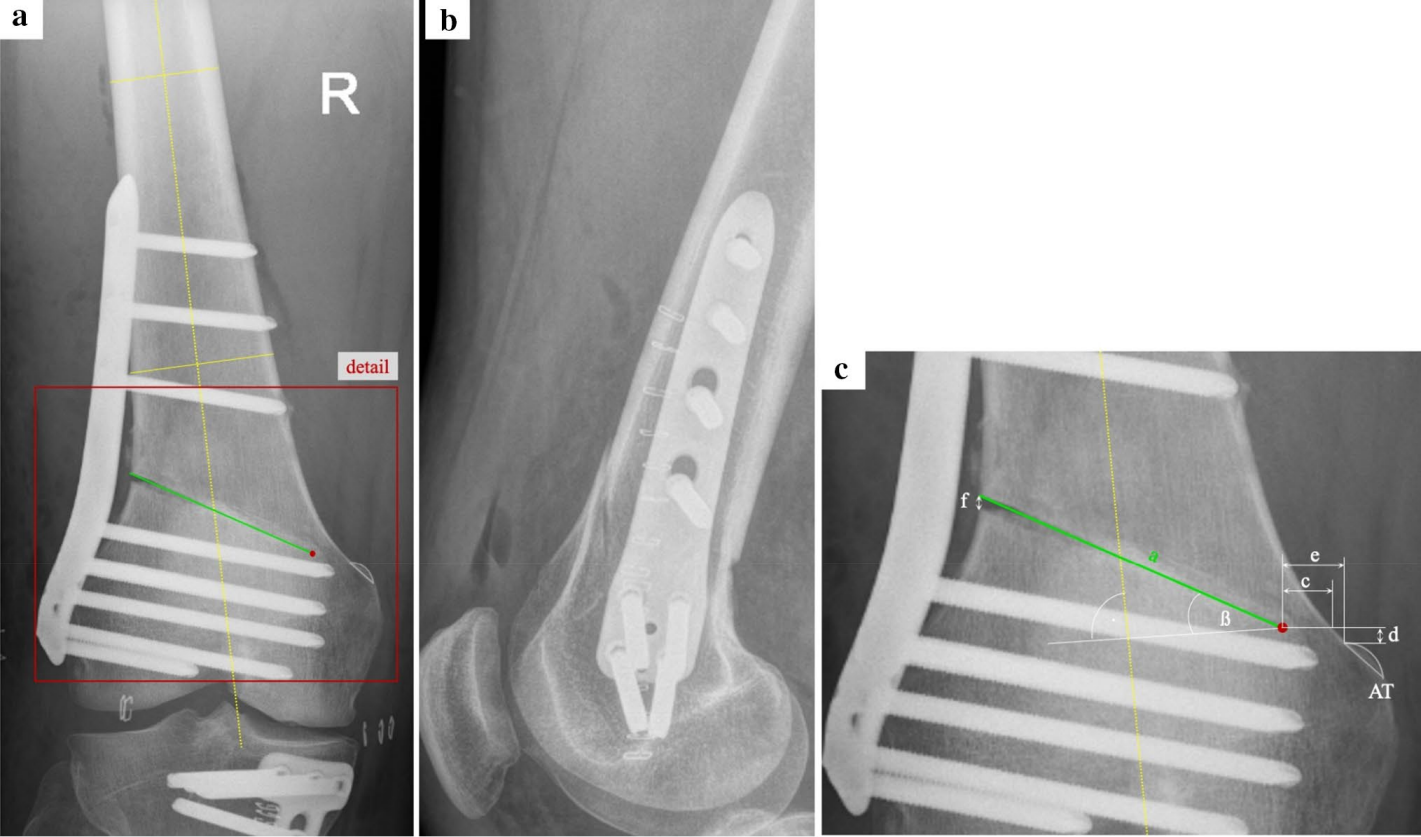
Measurement parameters. a Standard anterior–posterior and b lateral radiograph of a right knee after lateral closing wedge distal femoral osteotomy using a TomoFix™ (DePuy Synthes, Raynham, MA, USA) locking compression plate. c Detailed view of the closing wedge osteotomy cut with the respective measurements. AT, adductor tubercle (grey line); red dot, osteotomy hinge; yellow solid lines, mediolateral diameter femoral cortical bone; yellow dotted line, anatomical axis of the femoral diaphysis, running through the centre of the two yellow solid lines; green line (interval “a”), length of the osteotomy cut; interval “c”, horizontal distance between the medial cortical bone and the osteotomy hinge; interval “d”, vertical distance between the osteotomy hinge and the proximal border of the AT; interval “e”, horizontal distance between the osteotomy hinge and the proximal lateral margin of the AT; interval “f”, distance between the cortices proximal and distal of the osteotomy cut; angle “ß”, inclination of the osteotomy (angle between the proximal osteotomy plane and a line perpendicular to the anatomical axis of the femur)
